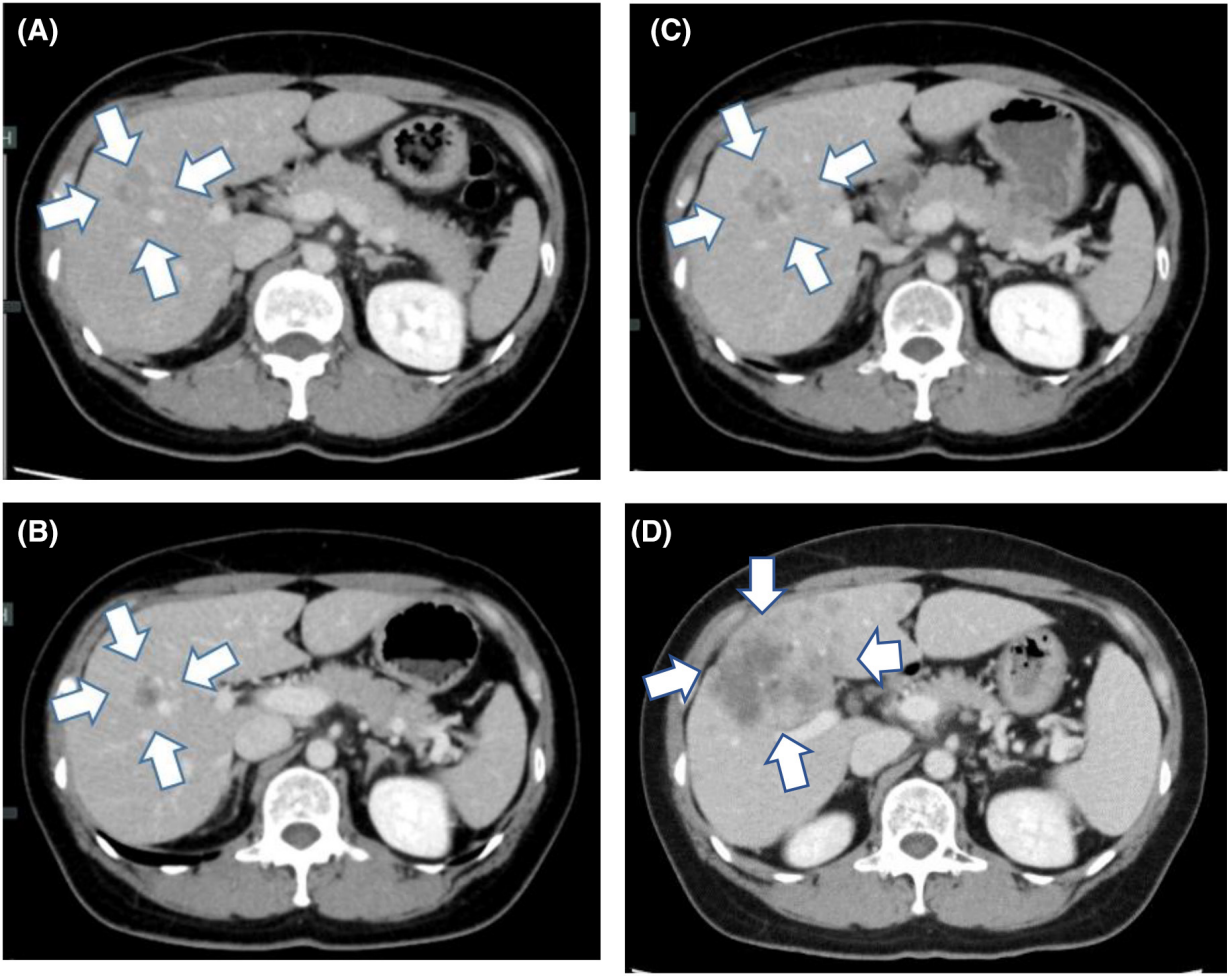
Liver metastasis on computed tomography. (A) Liver metastasis before olaparib administration (arrows). (B) Liver metastasis has shrunk (arrows). (C) Liver metastasis has progressed after 12 months of olaparib administration (arrows). (D) Liver metastasis (arrows) at the time of cancer genomic profiling testing.
